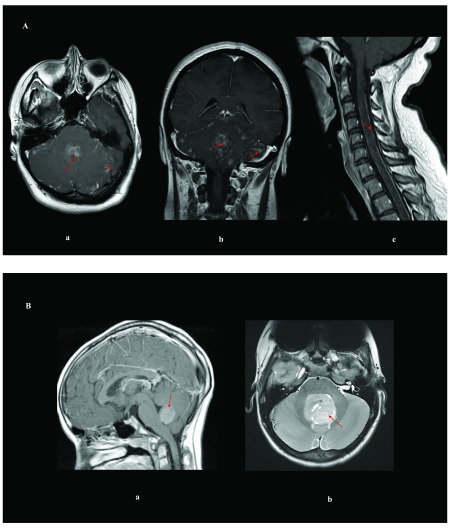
Imaging of pediatric and adult medulloblastomas. ( A) Magnetic resonance imaging of an adult woman who has
